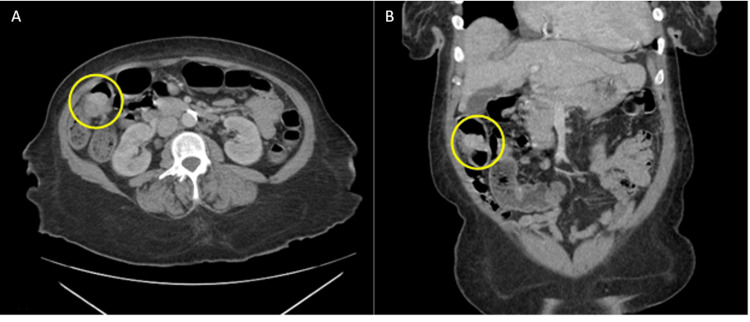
(A) CT imaging of the abdomen and pelvis in axial views demonstrating the cecal mass in question highlighted by the yellow circle. (B) CT imaging of the abdomen and pelvis in coronal views demonstrating the ileal mass in question highlighted by the yellow circle.
